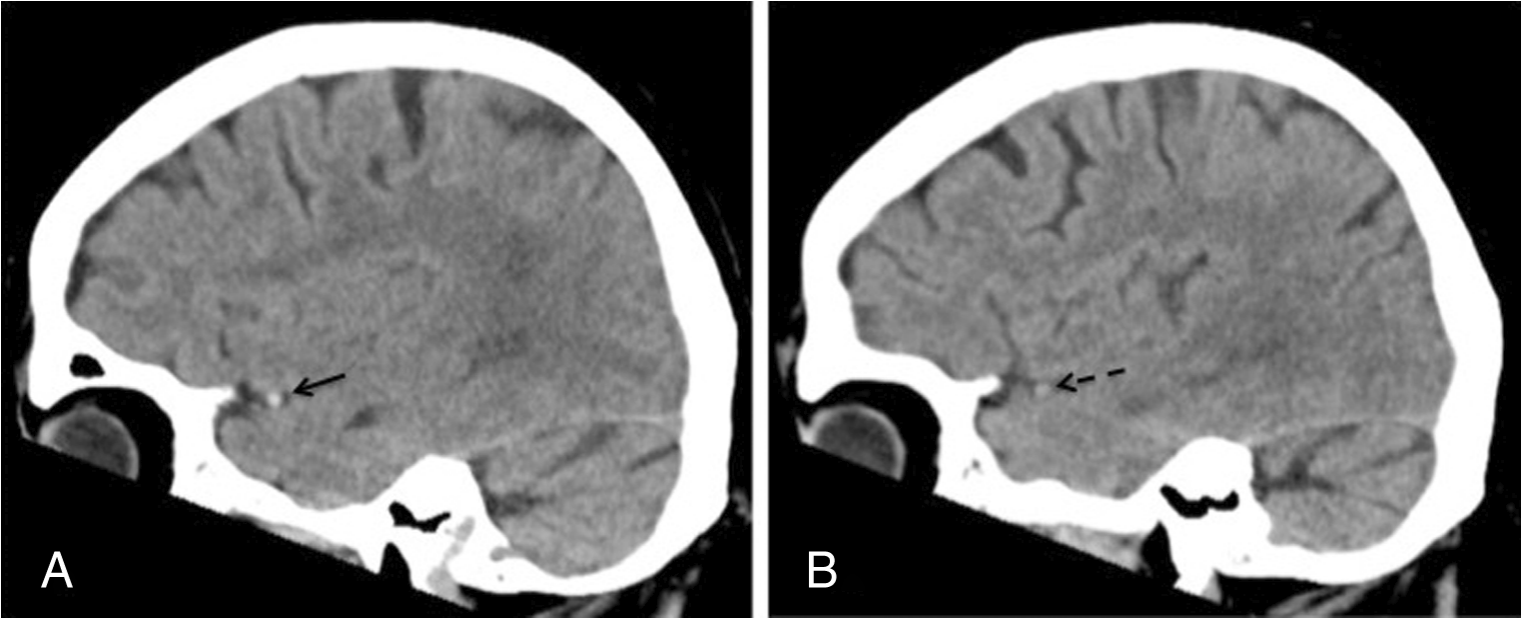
Sagittal reconstructions of a native CT scan of the same patient. a Thrombosis of MCA with a hyperdense sign (black arrow). b Freely perfused contralateral MCA (dashed arrow)
